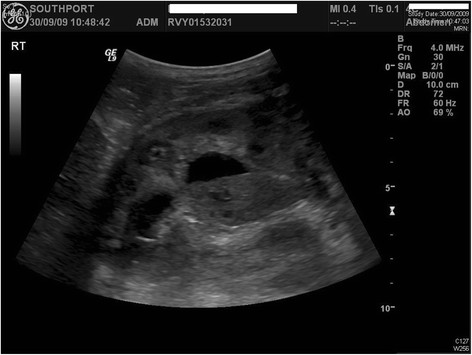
Ultrasound examination of urinary tract, performed when the patient was 39 years old: the right kidney was hydronephrotic with dilated renal pelvis. There was echogenic debris within the right collecting system.
